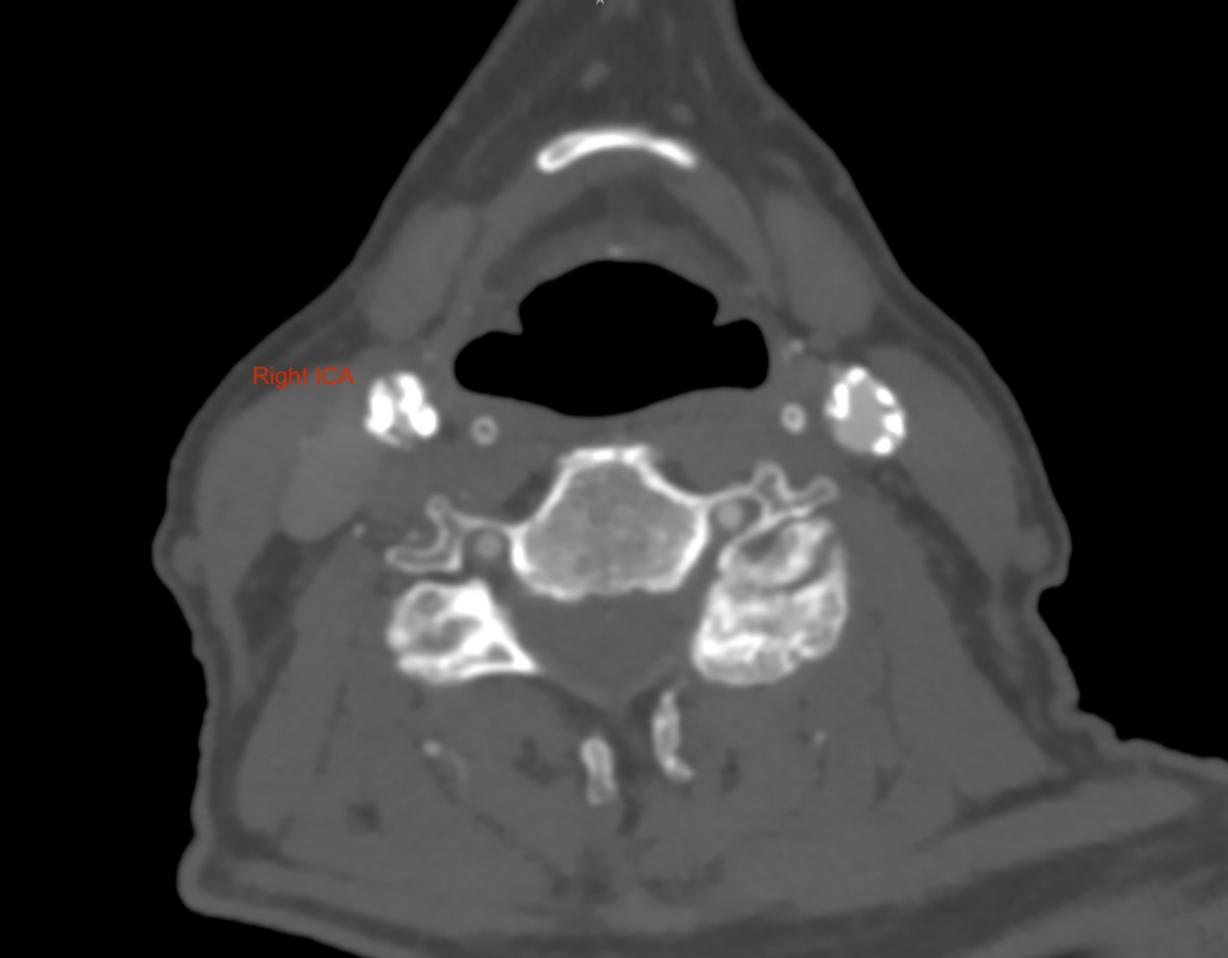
Computed tomography-angiogram (CT-A), axial section, demonstrating high grade stenosis of the right internal carotid artery (ICA).
